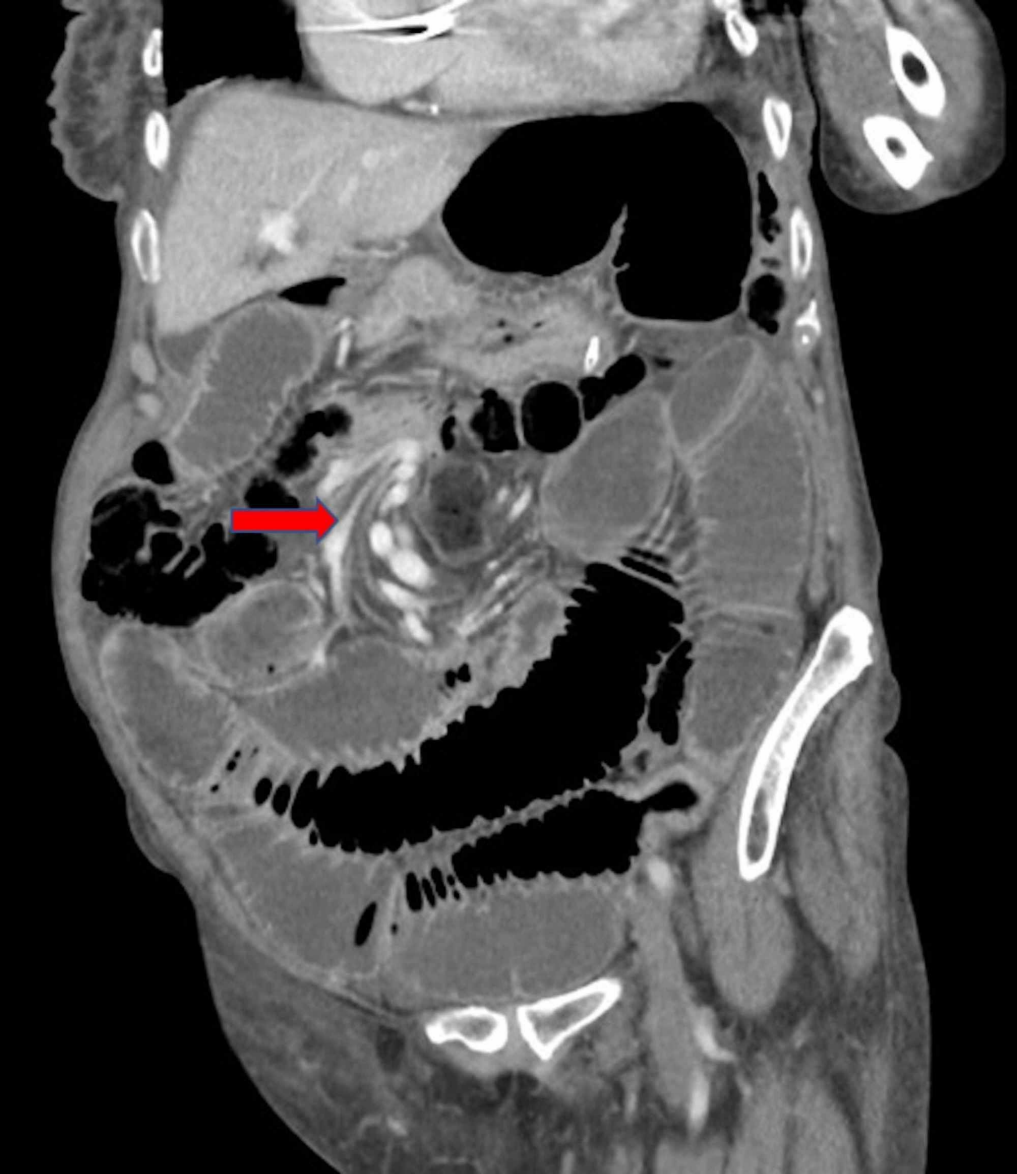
Coronal slice of abdominal computed tomography (CT) after contrast media administration, in portal phase demonstrating “whirlpool sign” of mesenteric vessels, suggestive of small bowel volvulus (arrow) with dilated loops of small bowel
